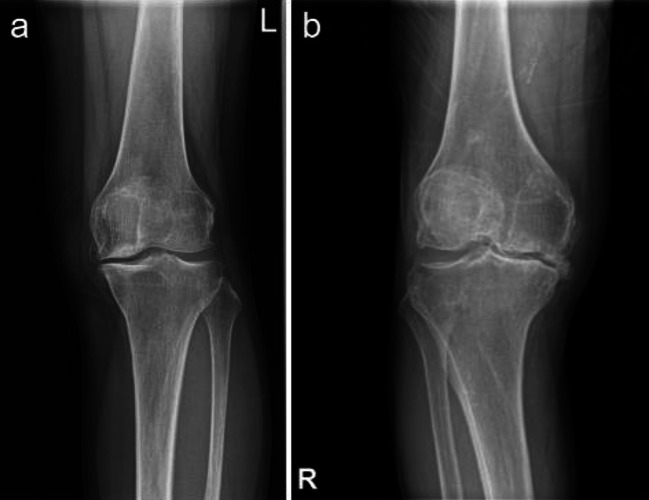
Plain X-ray of the knee, left one represents KL grade 3 a and right one represents KL grade 4 b
